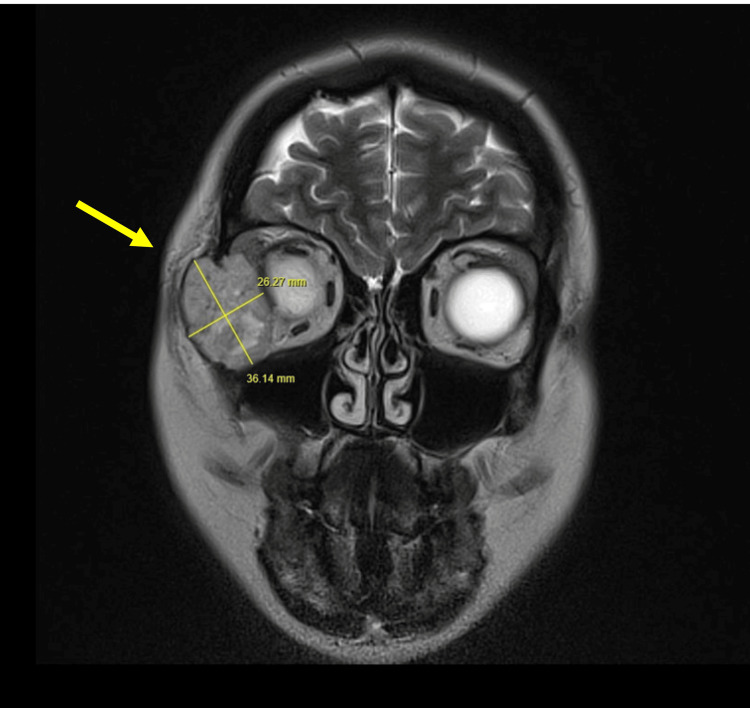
Coronal view of the MRI head with a well-defined mass (indicated by the arrow) at the right lateral orbit
